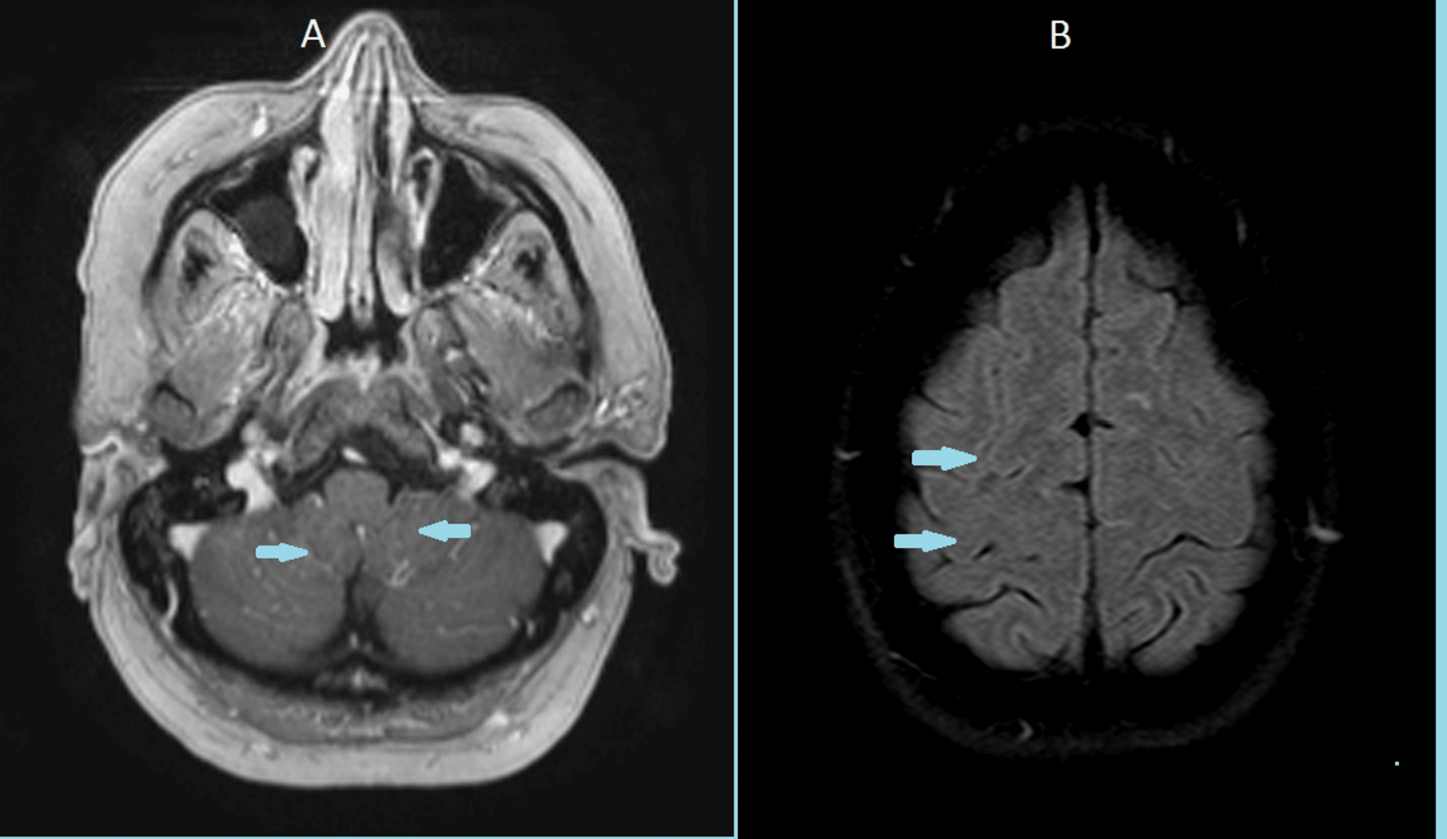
Brain magnetic resonance imaging demonstrates persistent meningeal enhancement in the posterior fossa, with minimal (nearly absent) enhancement in the cerebral hemispheres. Cortical and sulcal FLAIR hyperintensities have significantly decreased compared with the previous study, indicating radiological improvement (A: Axial plane (posterior fossa); B: Axial plane (supratentorial/cerebral hemispheres)) FLAIR: Fluid-attenuated inversion recovery
